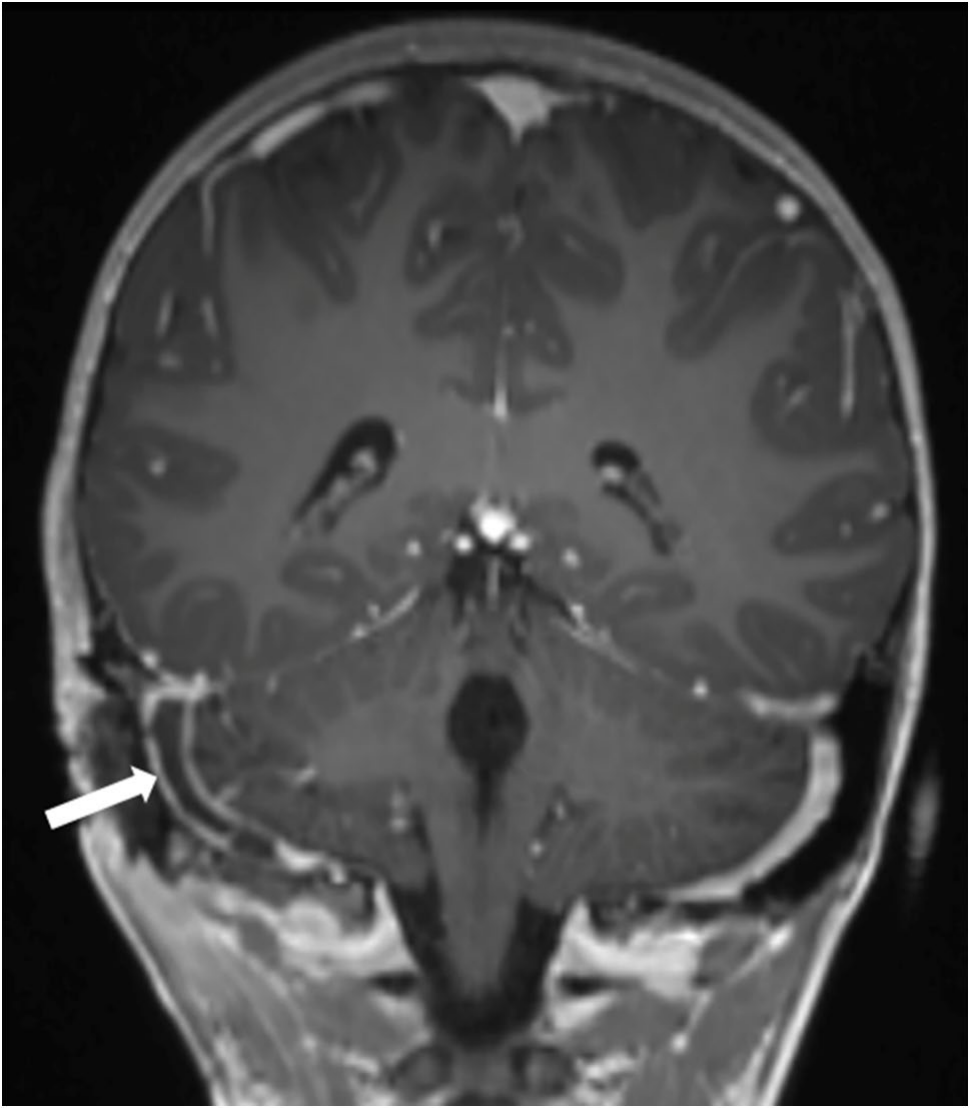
Coronal T1-weighted magnetization prepared-rapid gradient echo sequence magnetic resonance image after intravenous contrast agent administration in a 5-year-old girl with a sinus vein thrombosis in the right sigmoid sinus (*arrow*) caused by mastoiditis
